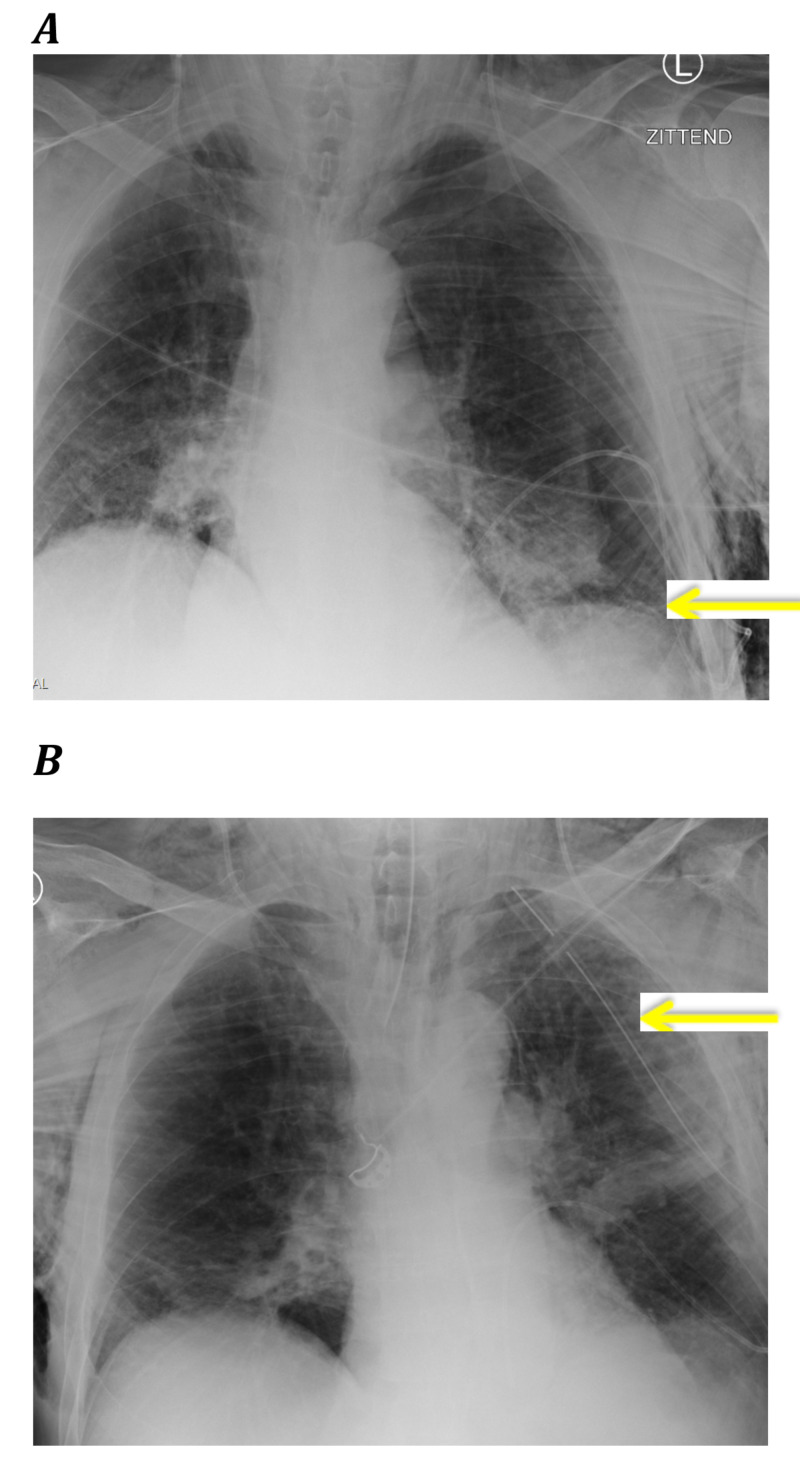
Chest X-rays directly after the surgery for the placement of the ventriculo-pleural drain, showing a pneumothorax of left lung. A: before thorax drain placement (the yellow arrow shows the pneumothorax) B: after thorax drain placement (the yellow arrow shows good placement of the thorax drain)
